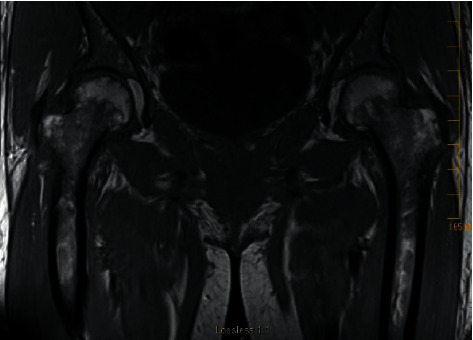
MRI pelvis revealed peripherally enhancing fluid collection extending into the adjacent soft tissues from the pubic symphysis consistent with septic arthritis. Left pubic body erosion without confluent marrow replacement indicates osteomyelitis. It also shows bilateral hip joint effusions with nonspecific left hip synovitis.
